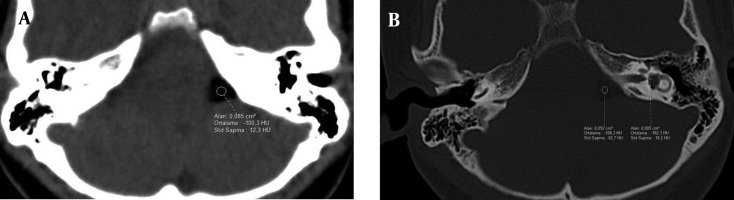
Axial CT images show lipoma as a hypodense lesion in A) the left cerebellopontine angle (HU of -100) and B) the left internal acoustic canal (HU of -182).
